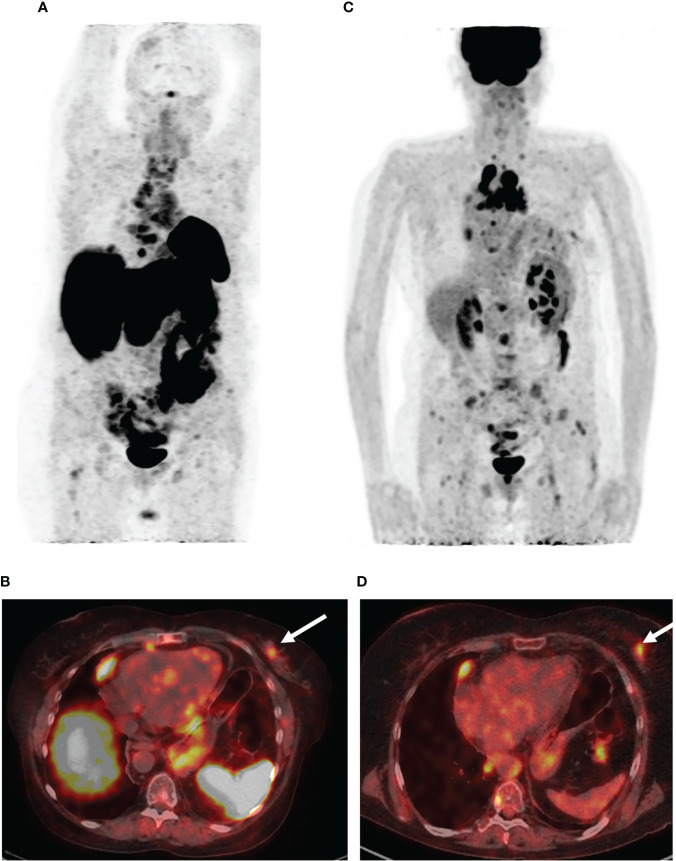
PET/CT images with [68Ga]-DOTA-TATE and [18F]-FDG. (A) The maximum intensity projection (MIP) images summarize the physiological and pathological distribution of [68Ga]-DOTA-TATE in the body. PET/CT scan showed an increase in the size of the previously detected metastases and the appearance of a new mediastinal lymph node and bone metastases. (B) [68Ga]-DOTA-TATE. PET/CT revealed an avid metastatic lesion in the upper inner quadrant of the left breast (white arrow). (C) The MIP images of [18F]-FDG PET showed intense tracer uptake in the same area as the [68Ga]-DOTA-TATE PET/CT scan. (D) [18F]-FDG-positive metastatic lesion in the left breast (white arrow).
